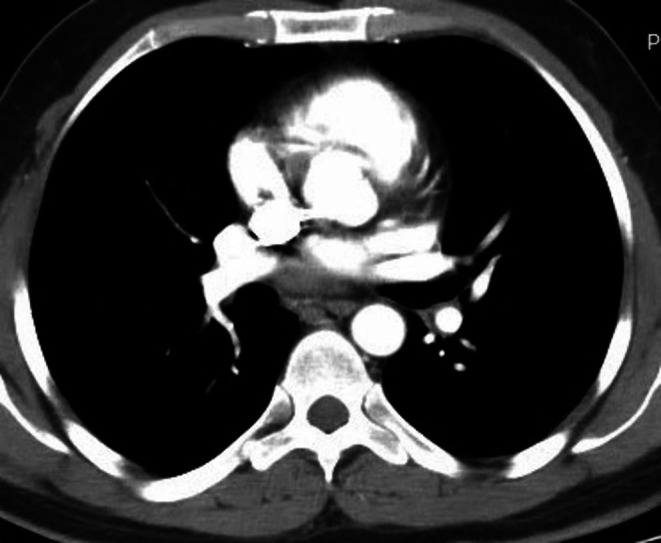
Chest CT after completion of TB treatment 21 February 2023 showing interval disappearance of the left side pleural effusion.
